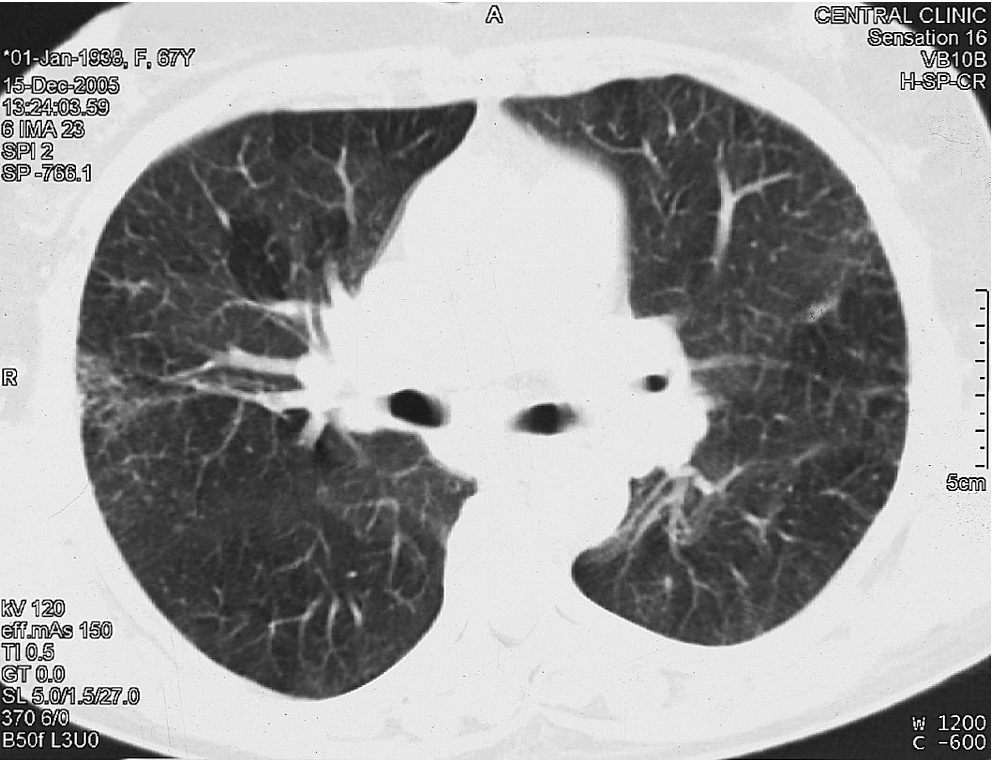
Computed tomography scan of the chest with bilateral mediastinal lymphadenopathy and bilateral interstitial lung opacities.
